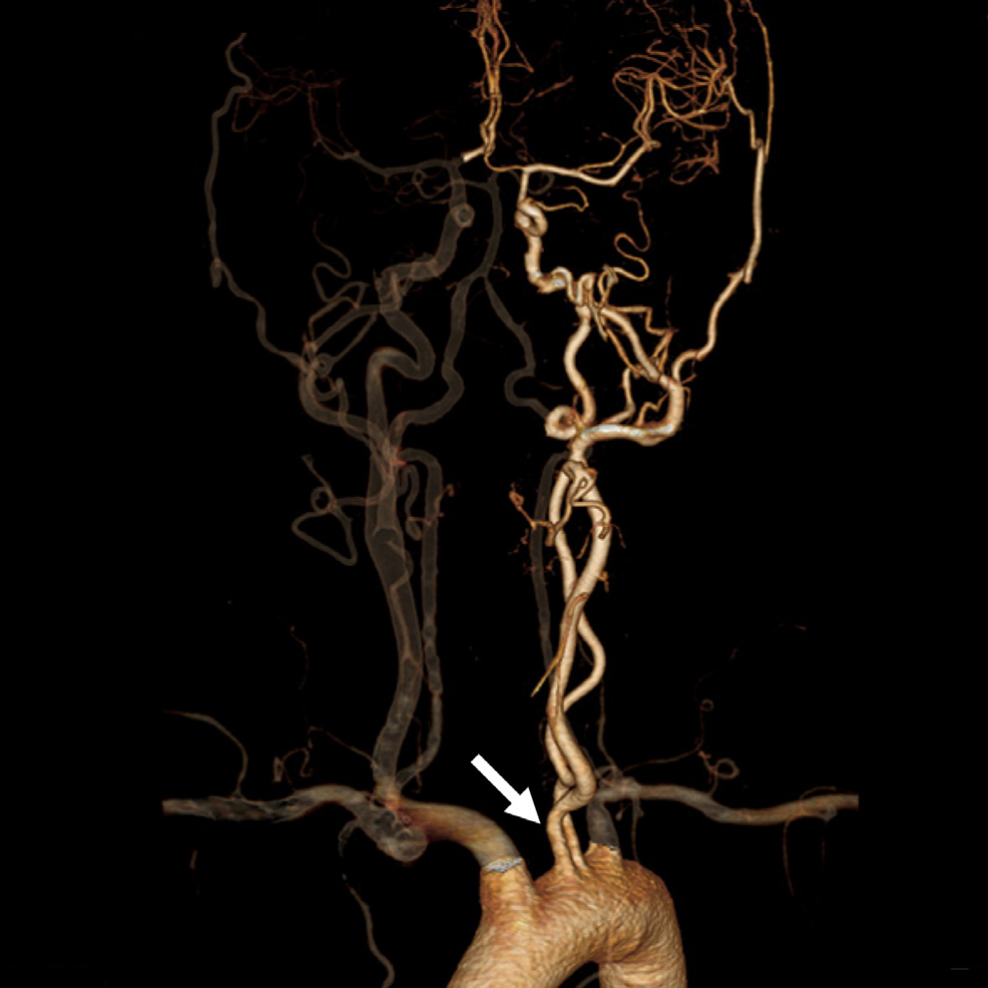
Computed tomography angiography demonstrating independent origins of the left internal and external carotid arteries from the aortic arch (arrow).
